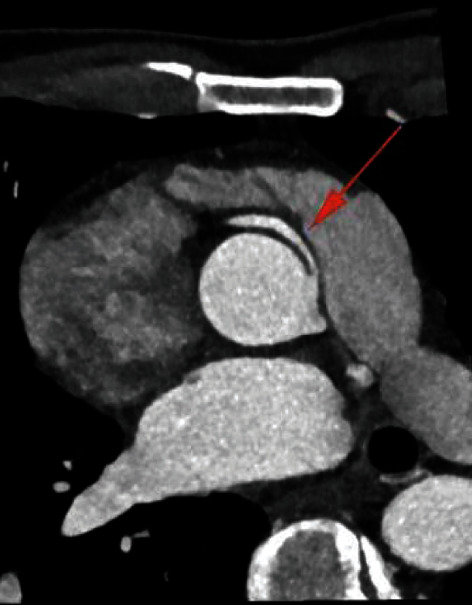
Coronary computed tomography showing the hypoplasic birth of right coronary artery (red arrow) originating close to the left sinus of Valsalva with further high-risk path between the aorta and the pulmonary artery.
